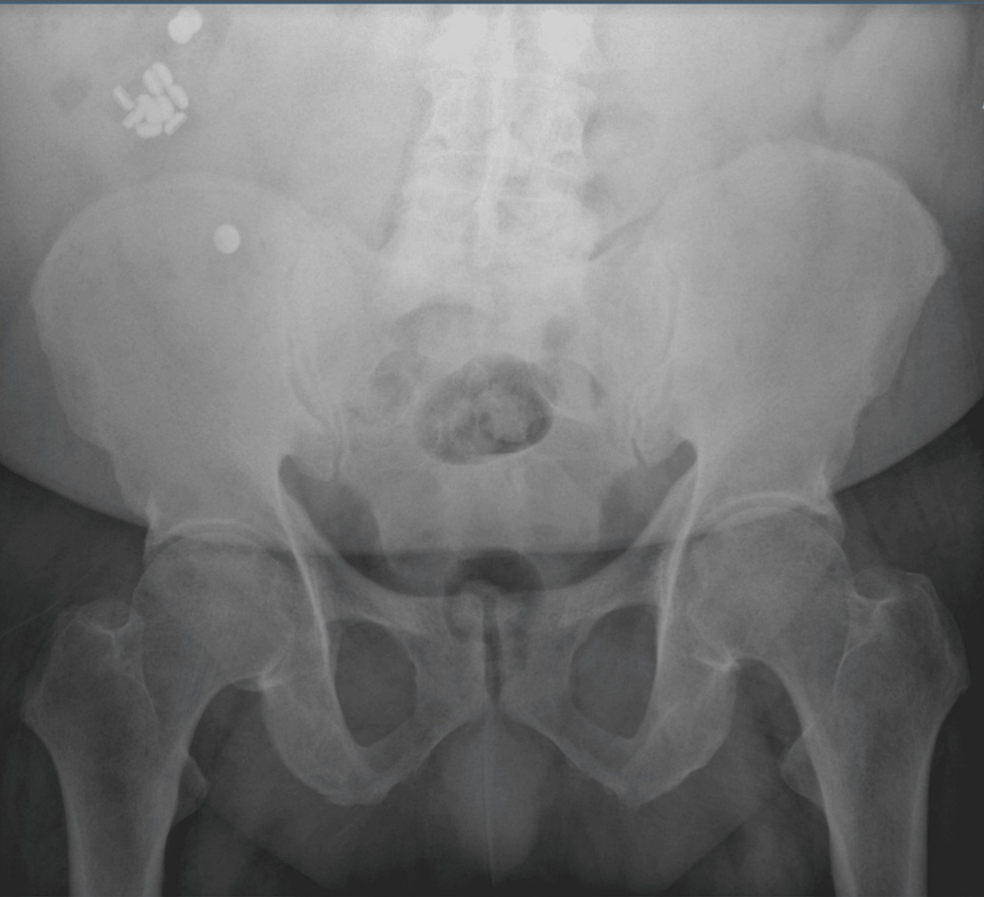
Multiple round hyperdense objects in the intestines on the radiograph
